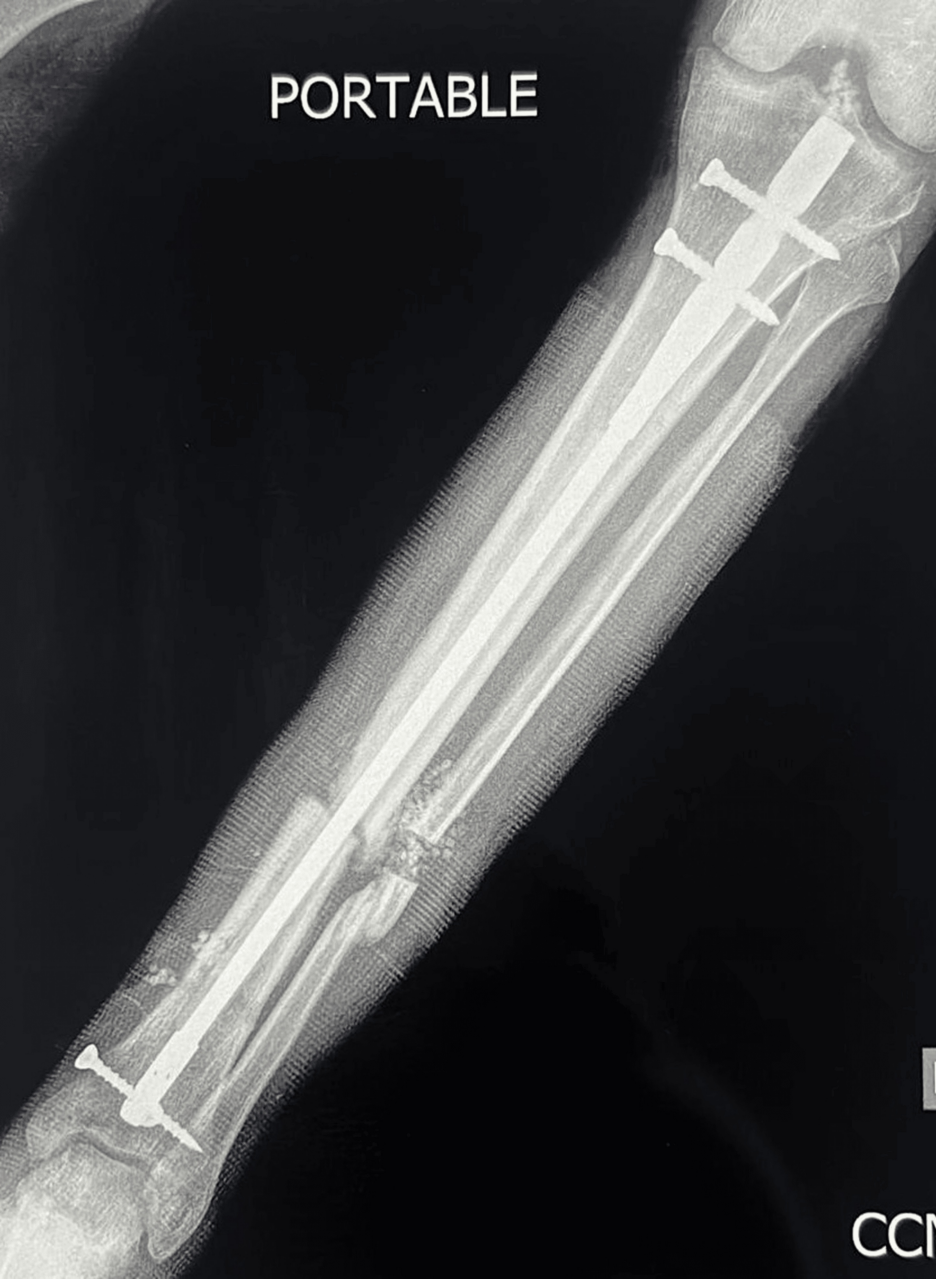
Postoperative X-ray of the left tibia and fibula AP view showing exchange nailing with a solid intramedullary nail with cement coating. AP: anterior posterior
